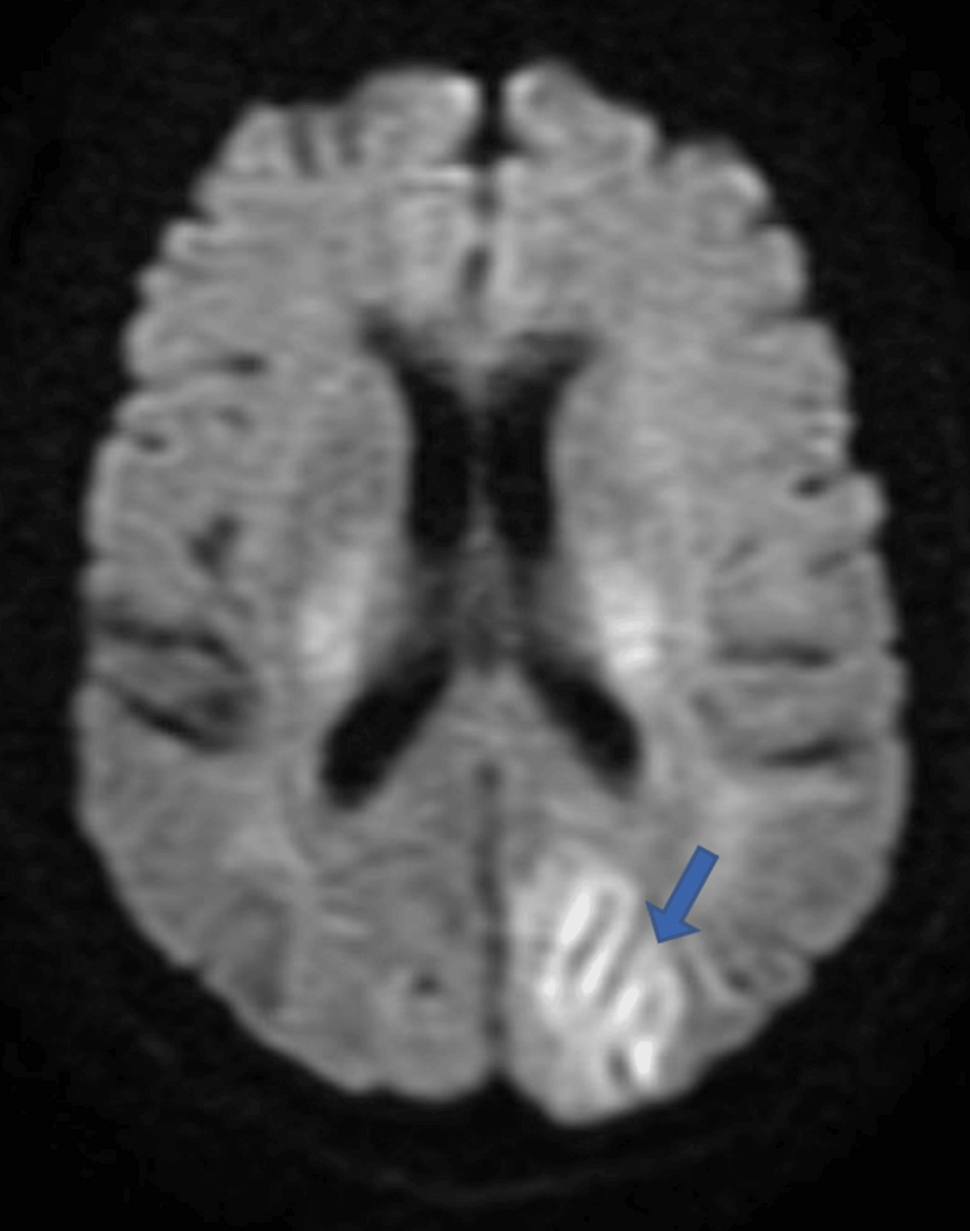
MRI brain showing few areas of diffusion restriction which were of high intensity on DWI sequence predominantly on the left side with gyriform pattern DWI: diffusion-weighted imaging
